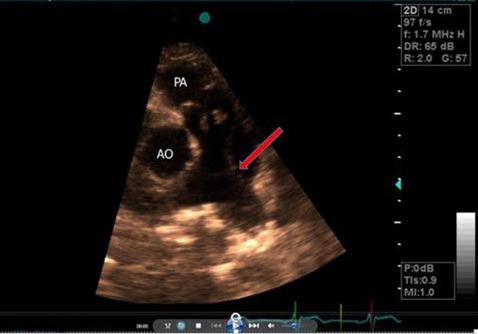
short axis parasternal view showing thickened and dome shaped pulmonary valve and intimal flap in lateral wall of main pulmonary artery (Red arrow).
